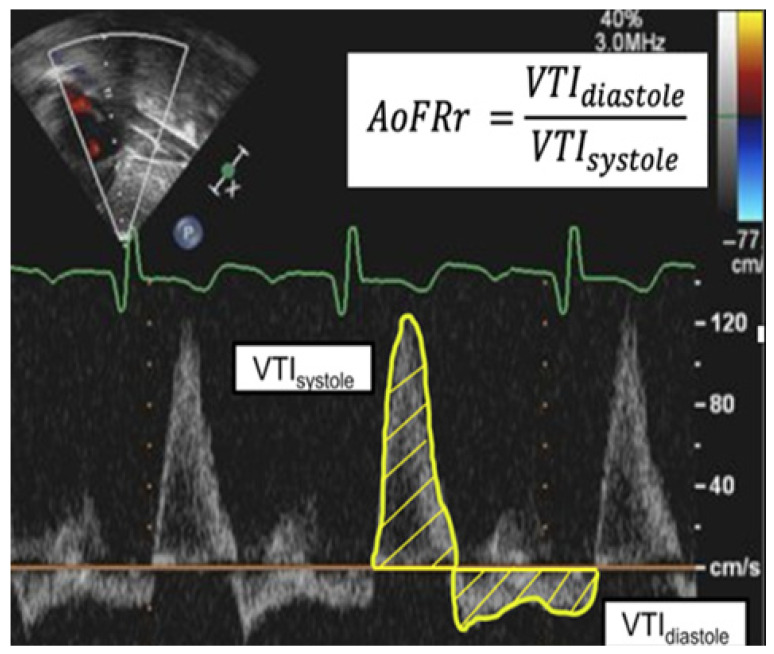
Echocardiographic Quantification of the Aortic Flow Reversal Ratio (AoFRr). A pulse-wave Doppler tracing from the abdominal aorta is used to calculate the AoFRr. The Velocity Time Integral (VTI), which is proportional to stroke volume, is measured by tracing the Doppler waveform. The systolic VTI (VTIsystole) represents the anterograde flow during systole (waveform above the baseline), while the diastolic reversal VTI (VTIdiastole) represents the retrograde flow stolen by the malformation during diastole (waveform below the baseline). The AoFRr is calculated as the ratio of these two values.
